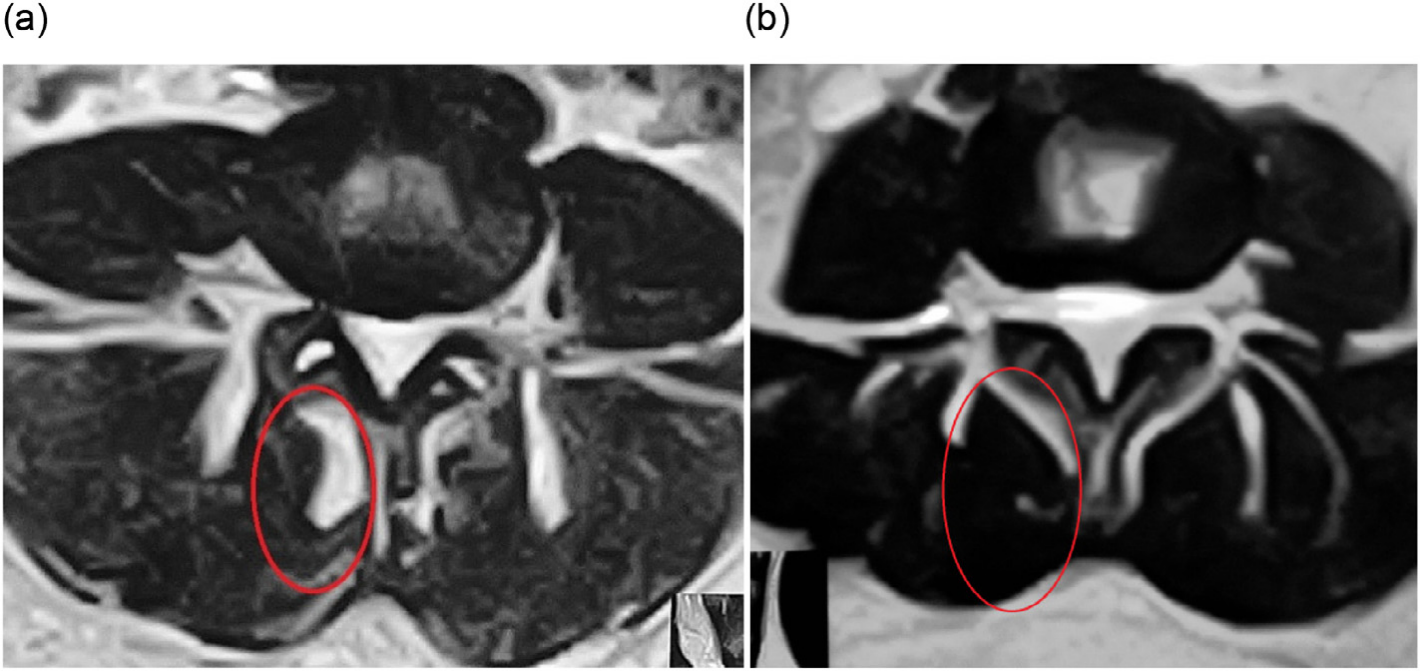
(a) Axial view of lumbar spine MRI shows severe multifidus atrophy, fatty degeneration (high signal intensity) is nearly 50% (red circle), (b) axial view of the same patient after one year post-injection of PLRP into atrophied lumbar multifidus with decreased fatty degeneration.
